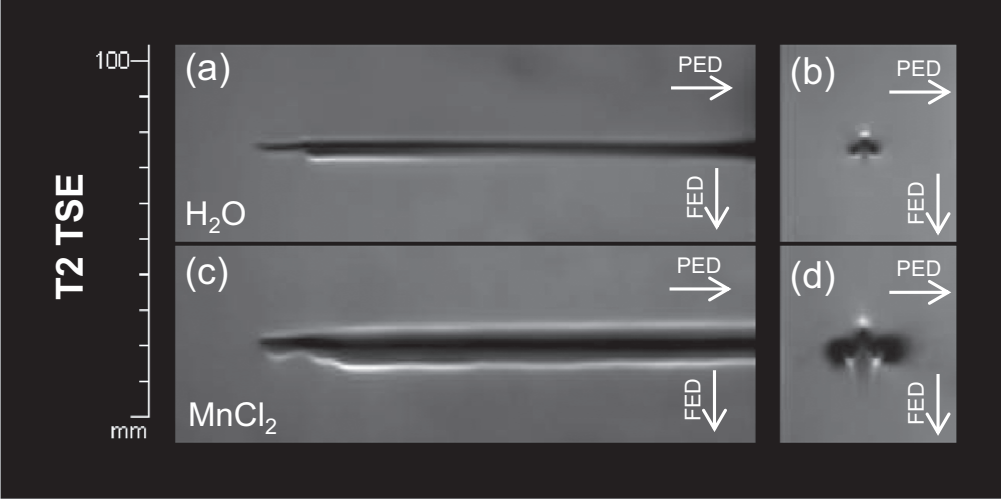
Artifact diameter of MRI compatible microwave therapeutic antennas can be enhanced by filling the cooling circulation system with fluid contrast agent instead of water. Angulation to B0 was 90°, (a) and (c) show coronal, (b) and (d) show axial slice orientation for the example of the T2 TSE sequence. The cooling circulation system was either filled with water (a, b) or 5 mmol/ml MnCl_2_ (c, d).
